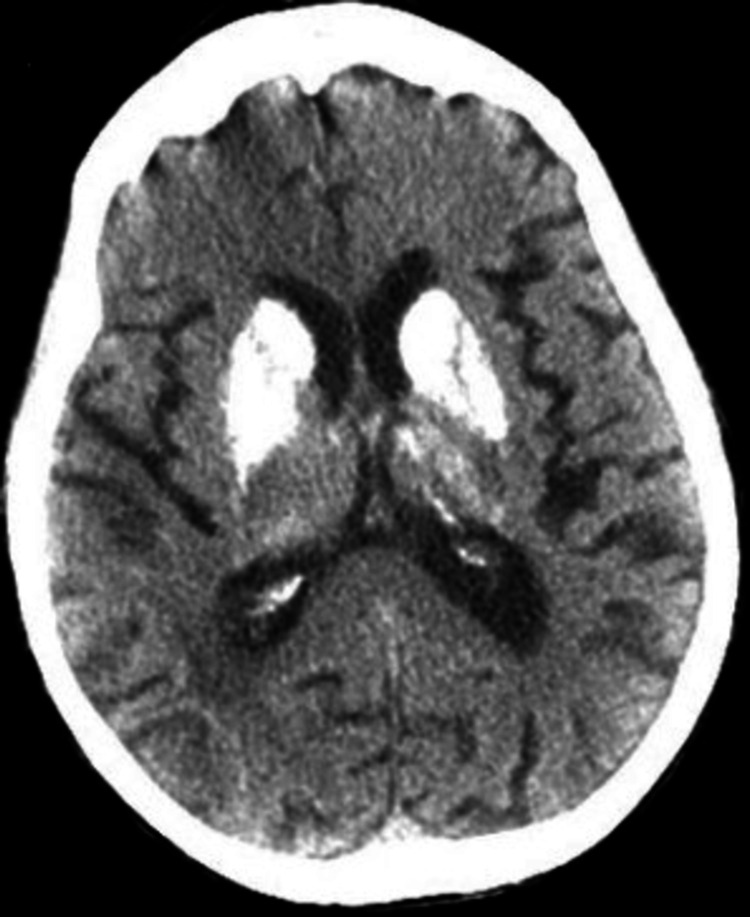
Non-Contrast CT of the brain, axial view This image shows bilateral calcification of the basal ganglia.
